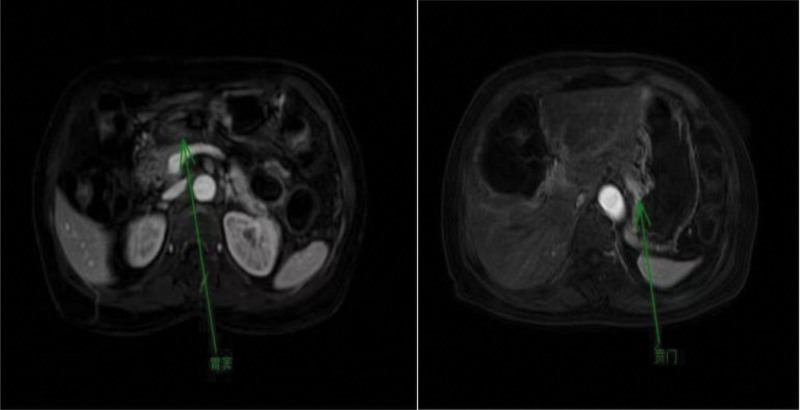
Plain scan of the upper abdomen + enhanced MRI indicated that there was local thickening and abnormal enhancement of the walls of the gastric cardia and antrum, without distant metastasis, which was consistent with the manifestations of gastric cancer (T2N1Mx). MRI = magnetic resonance imaging.
